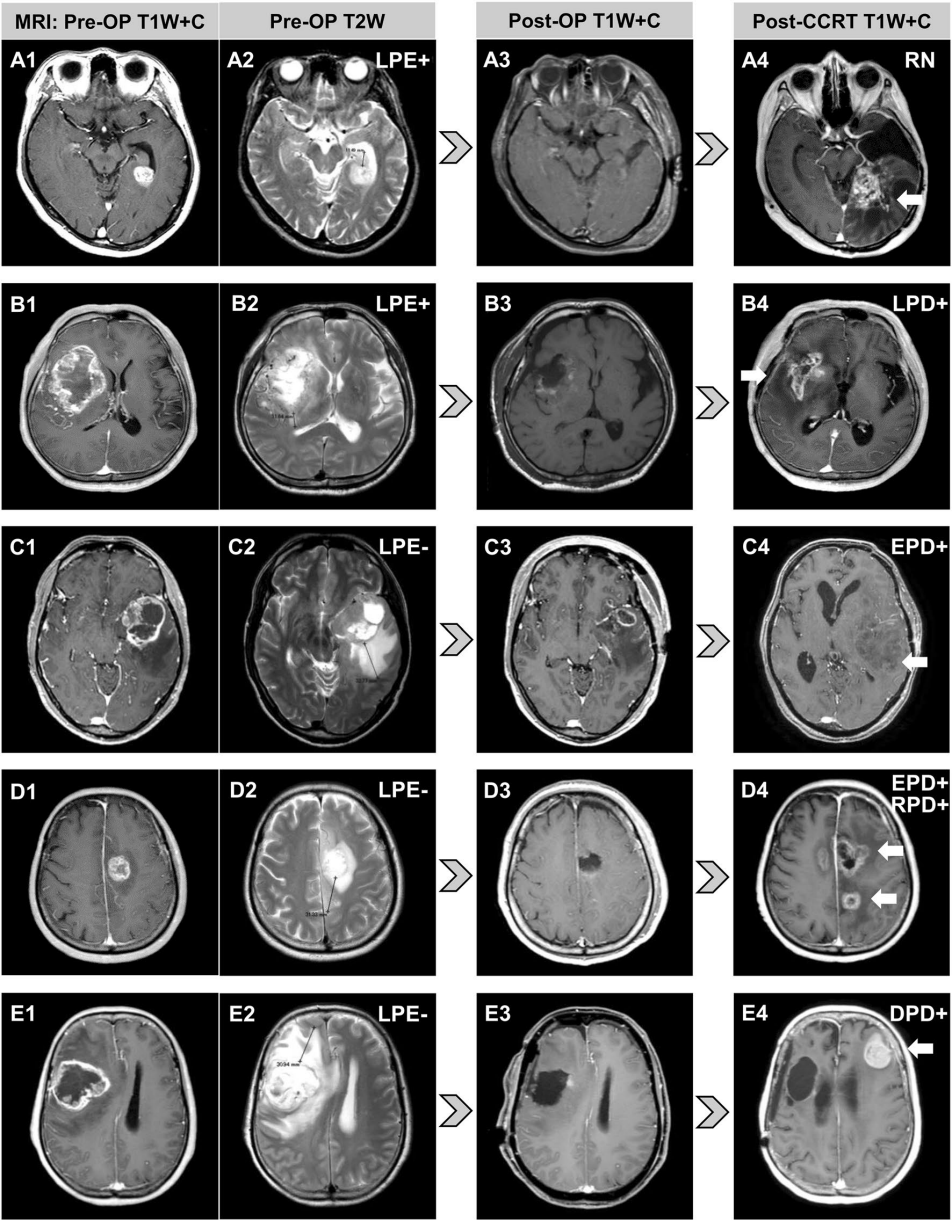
Diverse progression patterns observed after tumor resection and high-dose proton boost for five glioblastoma patients according to their peritumoral edema statuses. The arrows mark tumor progression. A LPE + patient with radiation necrosis only. B LPE + patient with tumor progression confined to the tumor bed. C LPE − patient with EPD. D LPE − patient with EPD, extending into the contralateral hemisphere and regional PD. E LPE − patient with distant tumor progression at the contralateral frontal lobe. *DPD* distant progressive disease, *EPD* extended progressive disease, *LPE* limited peritumoral edema, *OP* operative, *RN* radiation necrosis, *RPD* regional progressive disease, *T1W* + *C* contrast-enhanced T1-weighted magnetic resonance imaging
